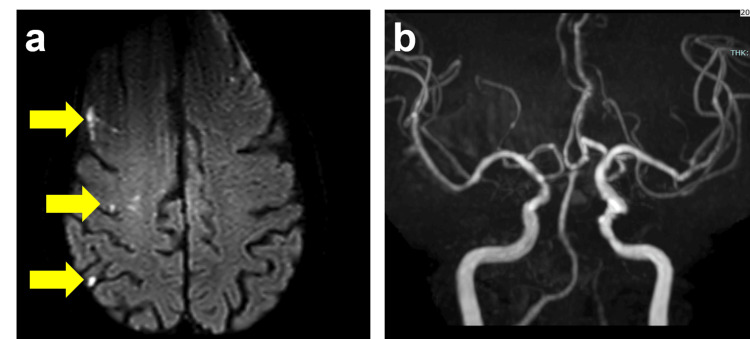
Postoperative findings a) Head MRI diffusion-weighted image reveals several asymptomatic acute cerebral infarctions in the right frontal and parietal subcortical white matter (arrows). b) Head MRA shows improved visualization of the right internal carotid artery and middle cerebral arteries.
